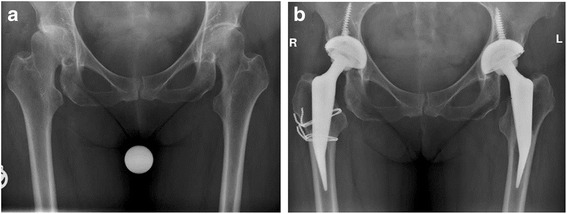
a Radiographs of a 49 year old patient with developmental dysplasia of the hip on both sides (b) Postoperative view after implantation of a Mayo conservative hip and a Harris Galante II cup (Zimmer). An undisplaced proximal femoral fracture occurred intraoperatively on the right side and was secured with 2 cerclage wires. A significant increased densitiy can be seen in zone 3
